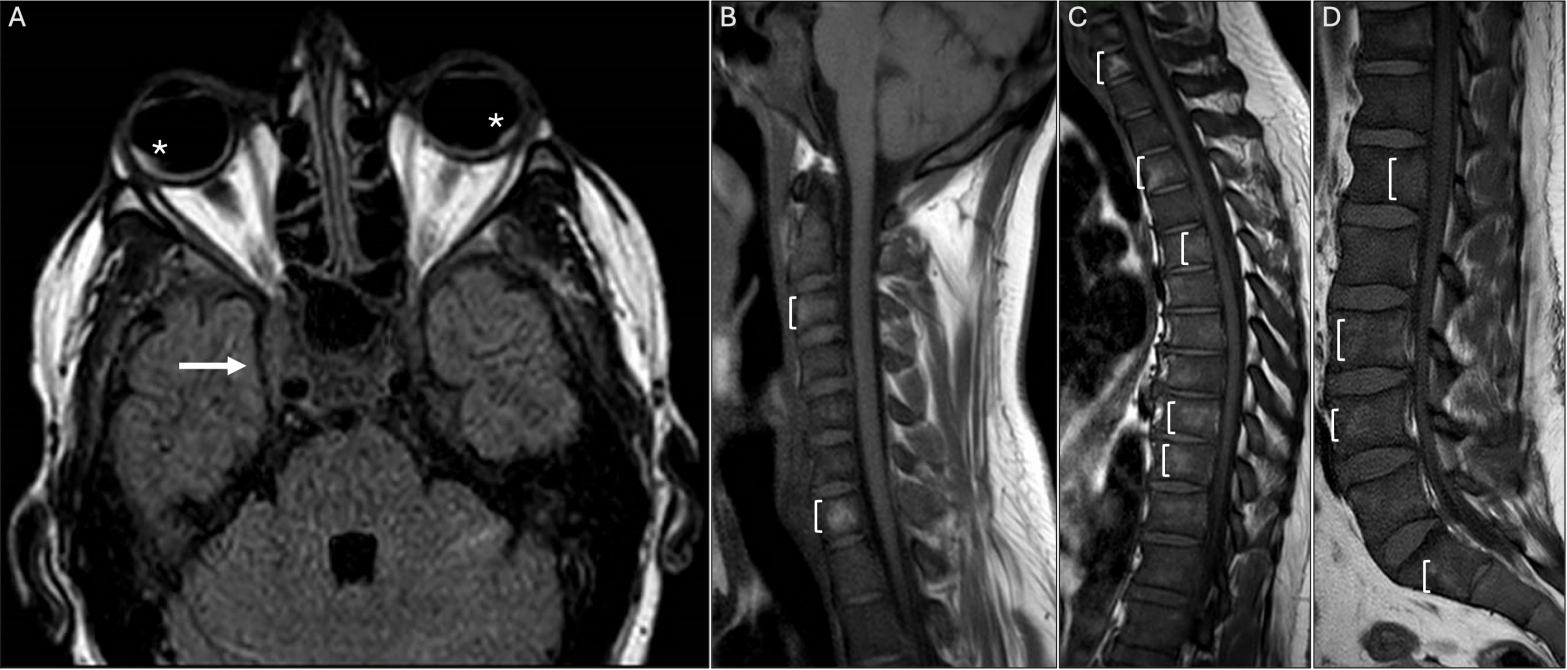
(A) Brain T2-weighted fluid-attenuated inversion recovery axial magnetic resonance image (MRI) demonstrates right-sided bulging of the cavernous sinus (arrow) and bilateral posterior intraocular hyperintensities corresponding to SRD (asterisk). (B-D) T1-weighted MRI shows heterogeneous bone marrow signal throughout the spine, involving the posterior elements, with diffuse low signal intensity on T1 (brackets); and high signal on T2, with patchy contrast enhancement (not shown).
